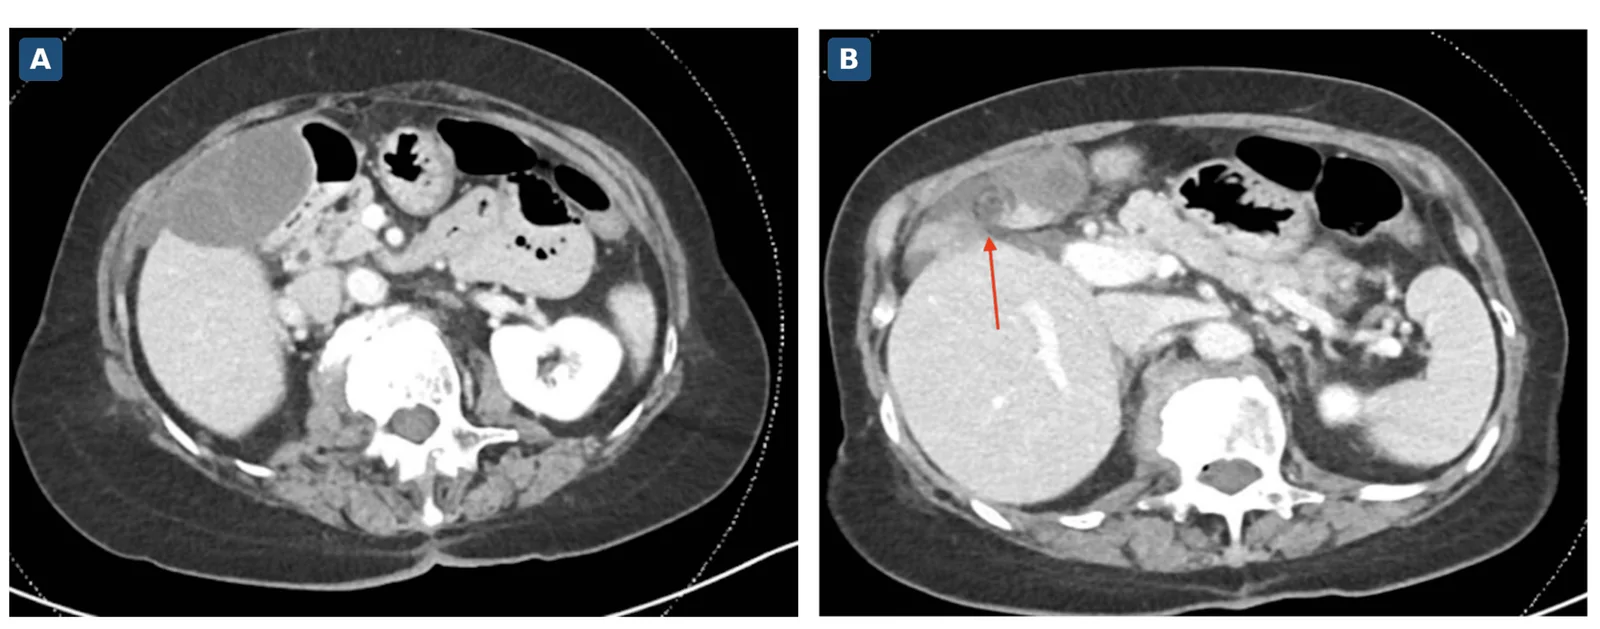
Intravenous contrast-enhanced abdominal computed tomography. (A) Distended gallbladder displaced from its normal fossa and situated anterior and lateral to the liver. (B) Twisting of the cystic pedicle, the whirl sign, is indicated by the red arrow, with reduced mural enhancement and wall irregularity.
